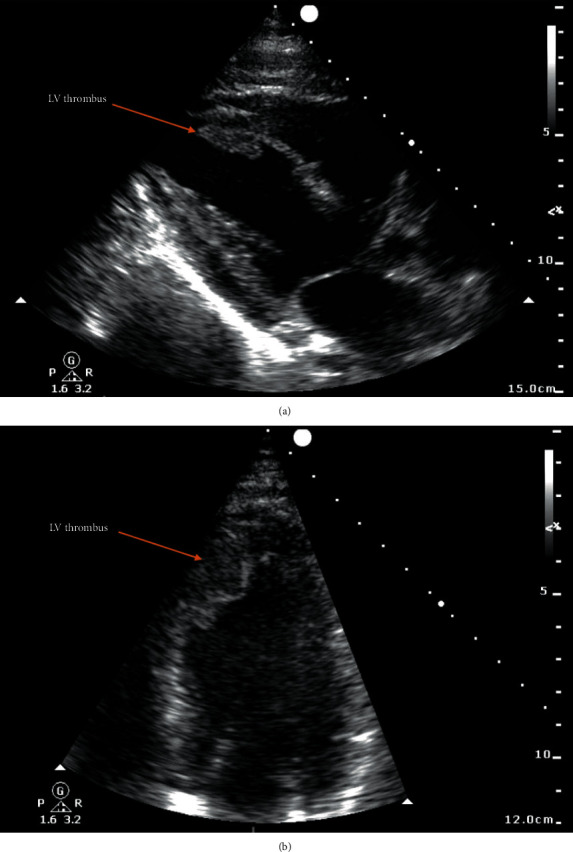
Transthoracic echocardiogram demonstrating thrombus on the mid-to-apical anteroseptal left ventricular wall in the (a) parasternal long axis view and (b) apical 4-chamber view.
